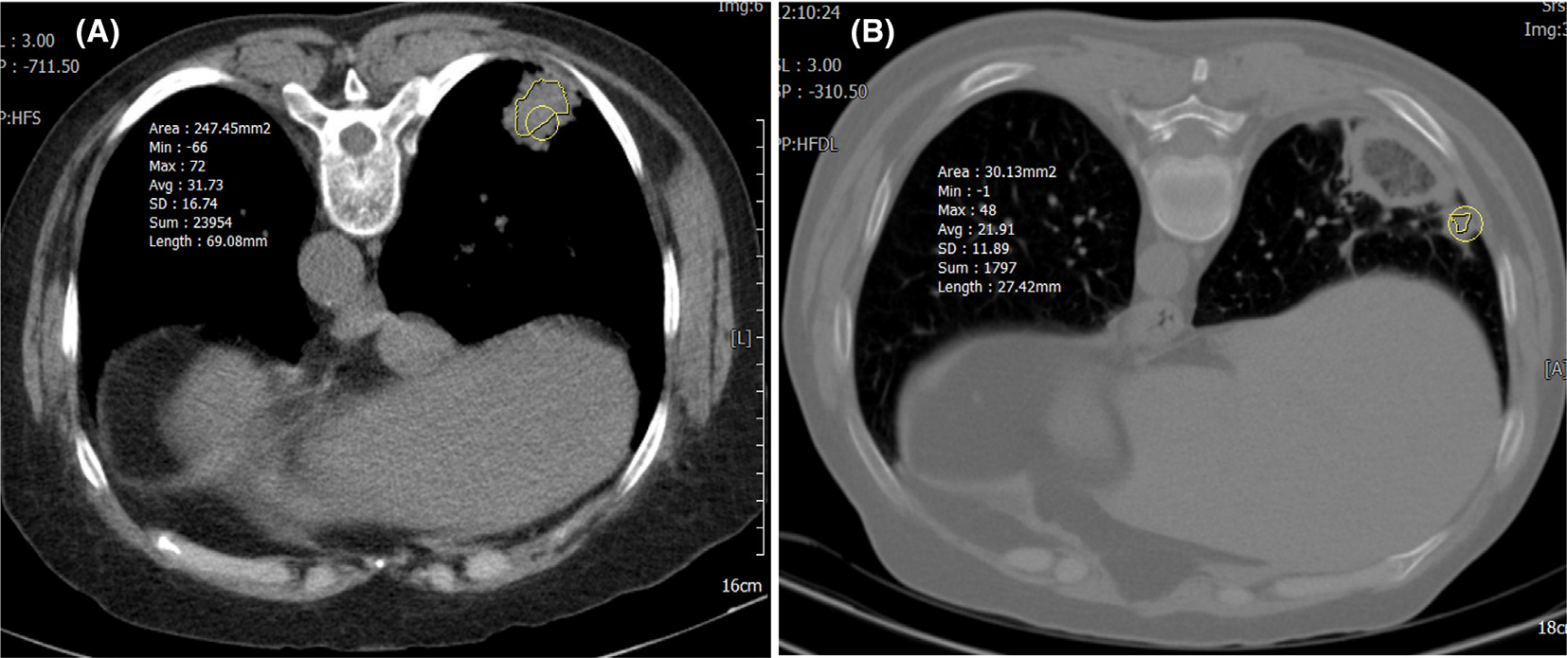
Unenhanced axial computed tomography (CT) image of (A) 72‐year‐old female having squamous cell carcinoma, (B) 41‐year‐old male having benign lesion, focal interstitial chronic inflammation.
